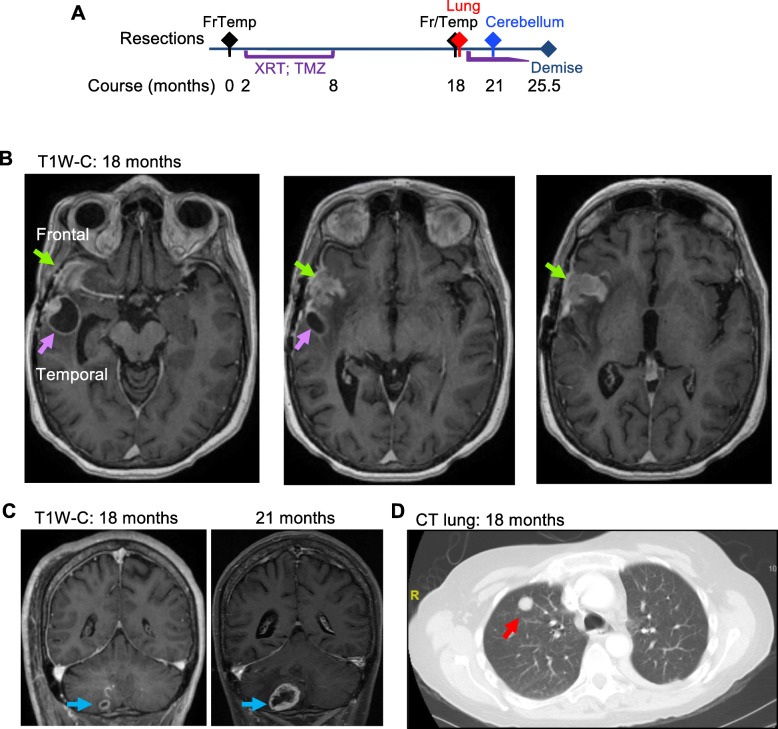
Clinical case presentation. a Time course of the patient’s clinical progression: the initial right frontotemporal (FrTemp) mass was surgically removed and the patient received standard radiotherapy (XRT) and temozolomide (TMZ). At 18 months postresection, 4 new ipsilateral masses occurred: frontal (Fr), temporal (Temp), as well as in the lung and cerebellum near midline, and underwent resections, followed by chemotherapy. b. MRI at the time of recurrence, 18 months following the 1st craniotomy for the initial mass resection. Serial axial T1W post-contrast (T1W-C) images show a multifocal mass comprising a frontal mass (Frontal, green arrows) at the site of the previous resection, and a temporal mass (Temporal, purple arrows). c Coronal T1W post-contrast (T1W-C) images showing rapid growth of a cerebellar mass (blue arrows) between the 2nd and the 3rd craniotomies. d Chest CT with contrast showing a solid mass in the right lung (red arrow)
